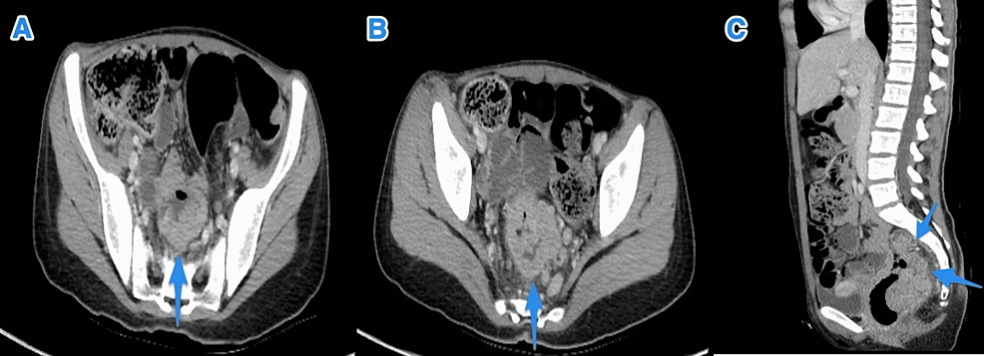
Baseline CT scan of the pelvis. CT scan of the pelvis axial (A and B) and sagittal (C) shows a mid rectal non-uniform wall thickening with a fungating mass (small arrow) associated with regional lymph nodes. Distally there is a large polypoidal enhancing intraluminal mass (large arrow) with a small pedicle posteriorly. There are no distant metastases.
